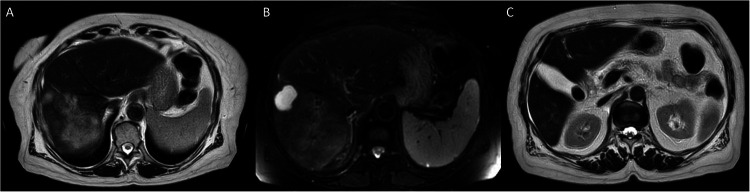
(A–C) Heterogeneous mass lesion in the right liver lobe, hyperintense on T2-weighted sequences.
